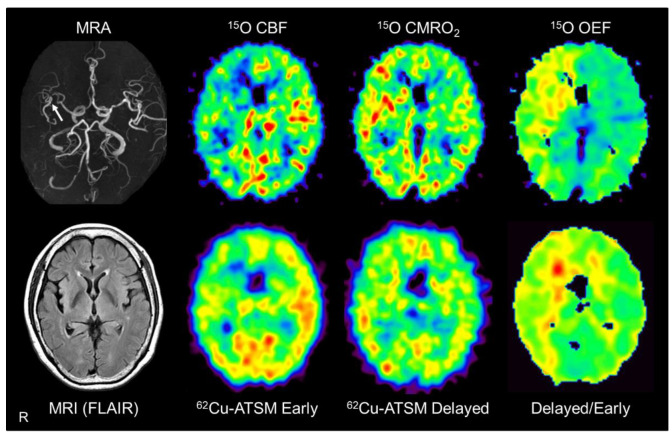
Brain positron emission tomography (PET) imaging with ^62^Cu-ATSM and ^15^O tracers (^15^O-water, ^15^O_2_, and C^15^O) in a representative patient with major cerebral arterial occlusive disease. Brain magnetic resonance imaging (MRI) showed severe stenosis in the right middle cerebral artery (arrow) but no apparent infarction. PET images with ^15^O tracers (upper images) showed decreased cerebral blood flow (CBF) with increased cerebral metabolic rate of oxygen (CMRO_2_) and oxygen extraction fraction (OEF) in the right cerebral hemisphere, which indicates misery perfusion in this area. Alongside ^15^O-gas and water PET, early, delayed, and delayed-to-early ratio images from ^62^Cu-ATSM PET (lower images) correspond well with the CBF, CMRO_2_, and OEF images, respectively. MRA: MR angiography; FLAIR: Fluid attenuation inversion recovery.
